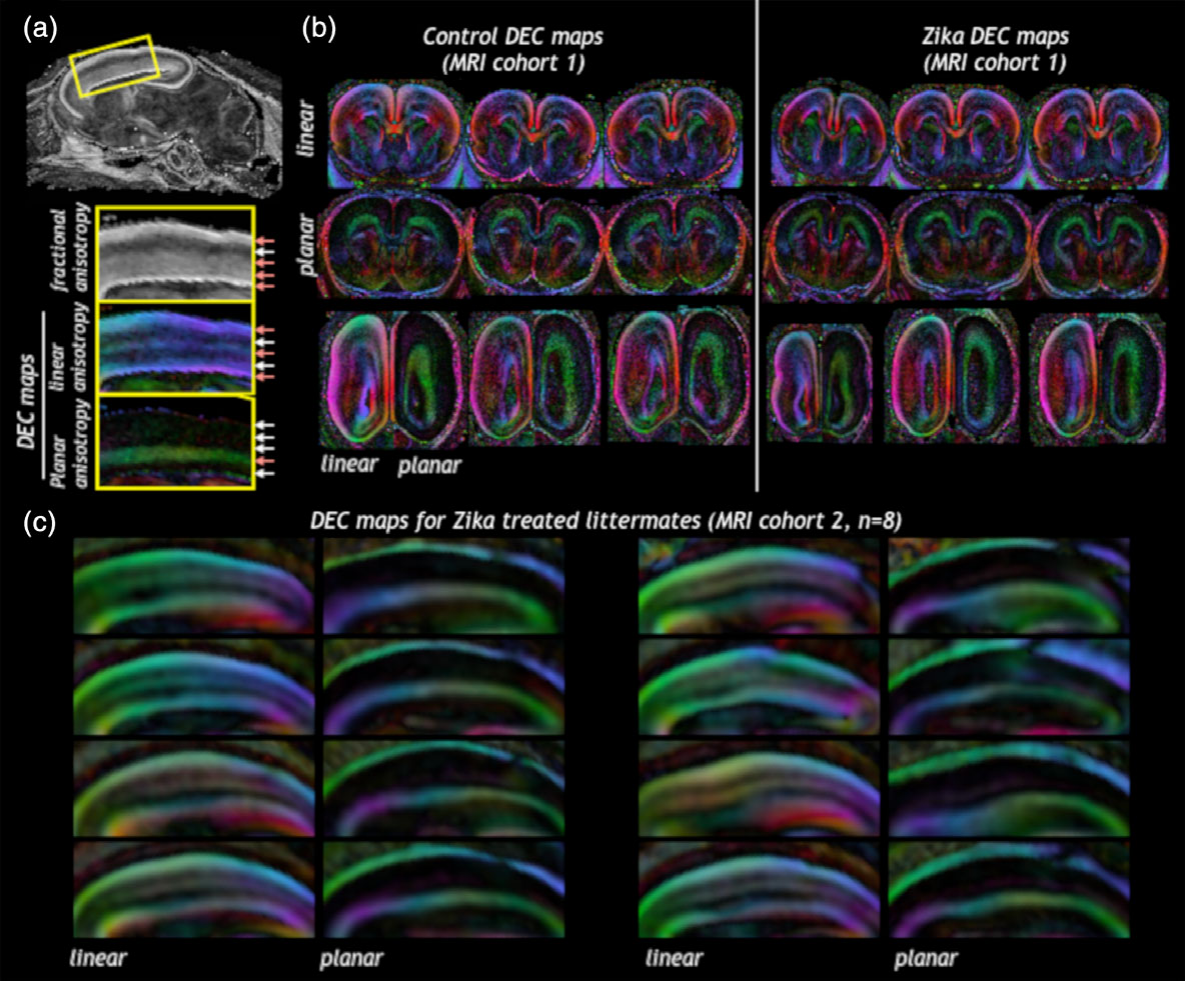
Cortical zones maintain their overall regional organization according to DTI features in control or Zika treated brains. Linear and planar anisotropy weighted directionally encoded color (DEC) maps were able to distinguish between different cortical zones (a) according to intensity and color of the metric. The presence and organization of cortical zones as indicated by DEC maps were similar in brains from Zika infected litters as from controls (b, MRI cohort 1 and c, MRI cohort 2). Note: This analysis measured laminar organization only, for volume analysis see Figure 7b
